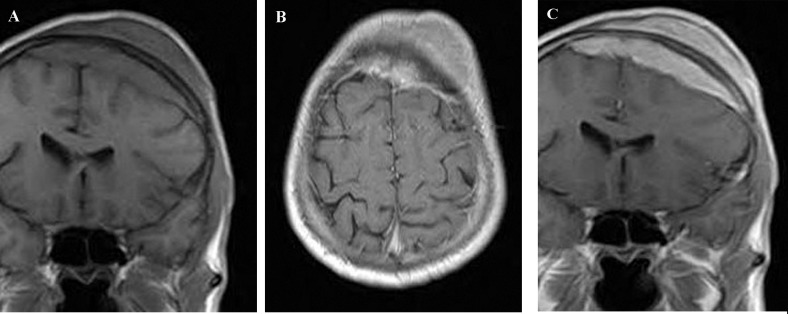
T1-weighted MR image before (A) and after (B,C) contrast medium reveals marked enhancement of the lesion and the dura beneath. Note also the almost normal marrow signal of the intervening skull in the regions without hyperostosis
